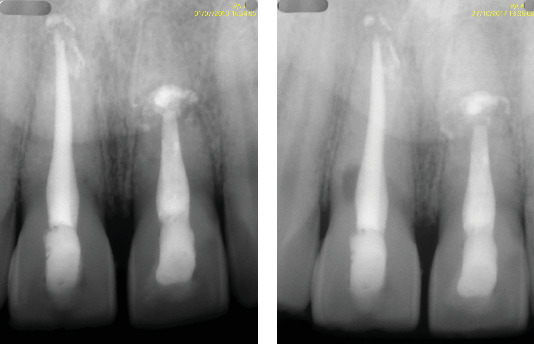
(a) The 10-year follow-up periapical radiograph shows no signs of pathology, (b) whereas the radiograph taken at 17 years shows evidence of resorption on the distal surface of the root.
